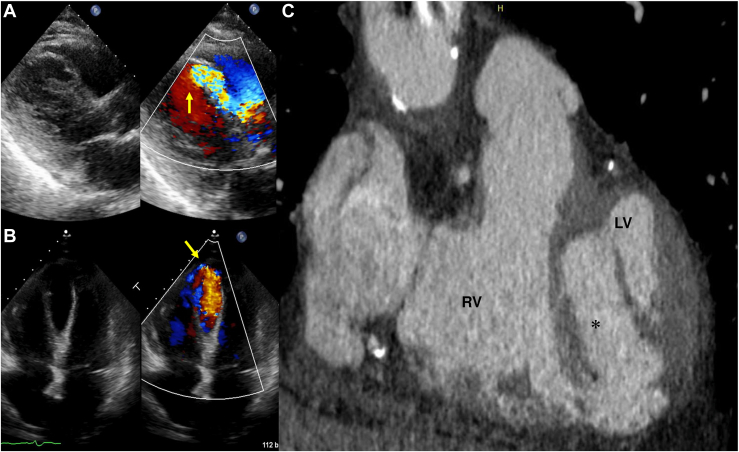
Transthoracic echocardiography demonstrating (A) a shunt (arrow) from the left ventricle (LV) to the dissected interventricular septum and (B) a shunt (arrow) from the dissected septum into the right ventricle (RV). (C) Contrast-enhanced computed tomography showing the communication between the LV and RV through the dissected interventricular septum (asterisk).
